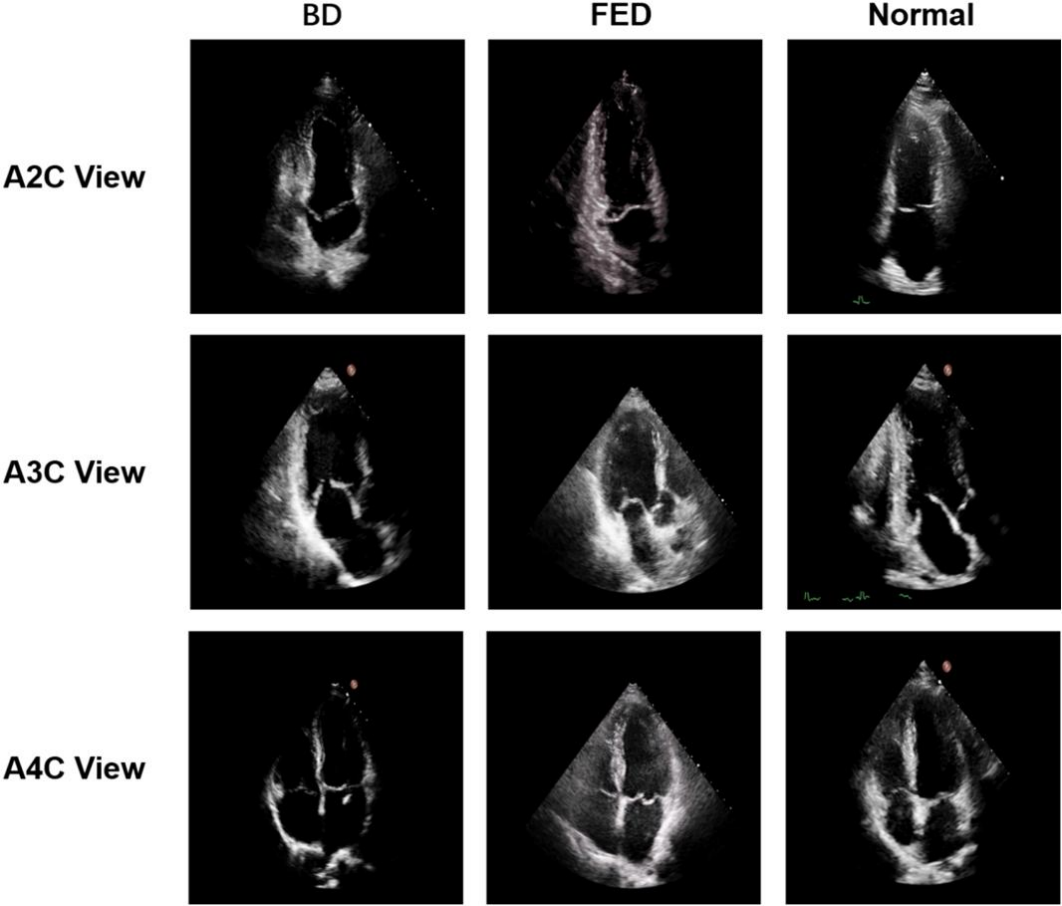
The image presents a sample echocardiographic image of the different types of MVP and healthy controls in A2C, A3C, and A4C views.
